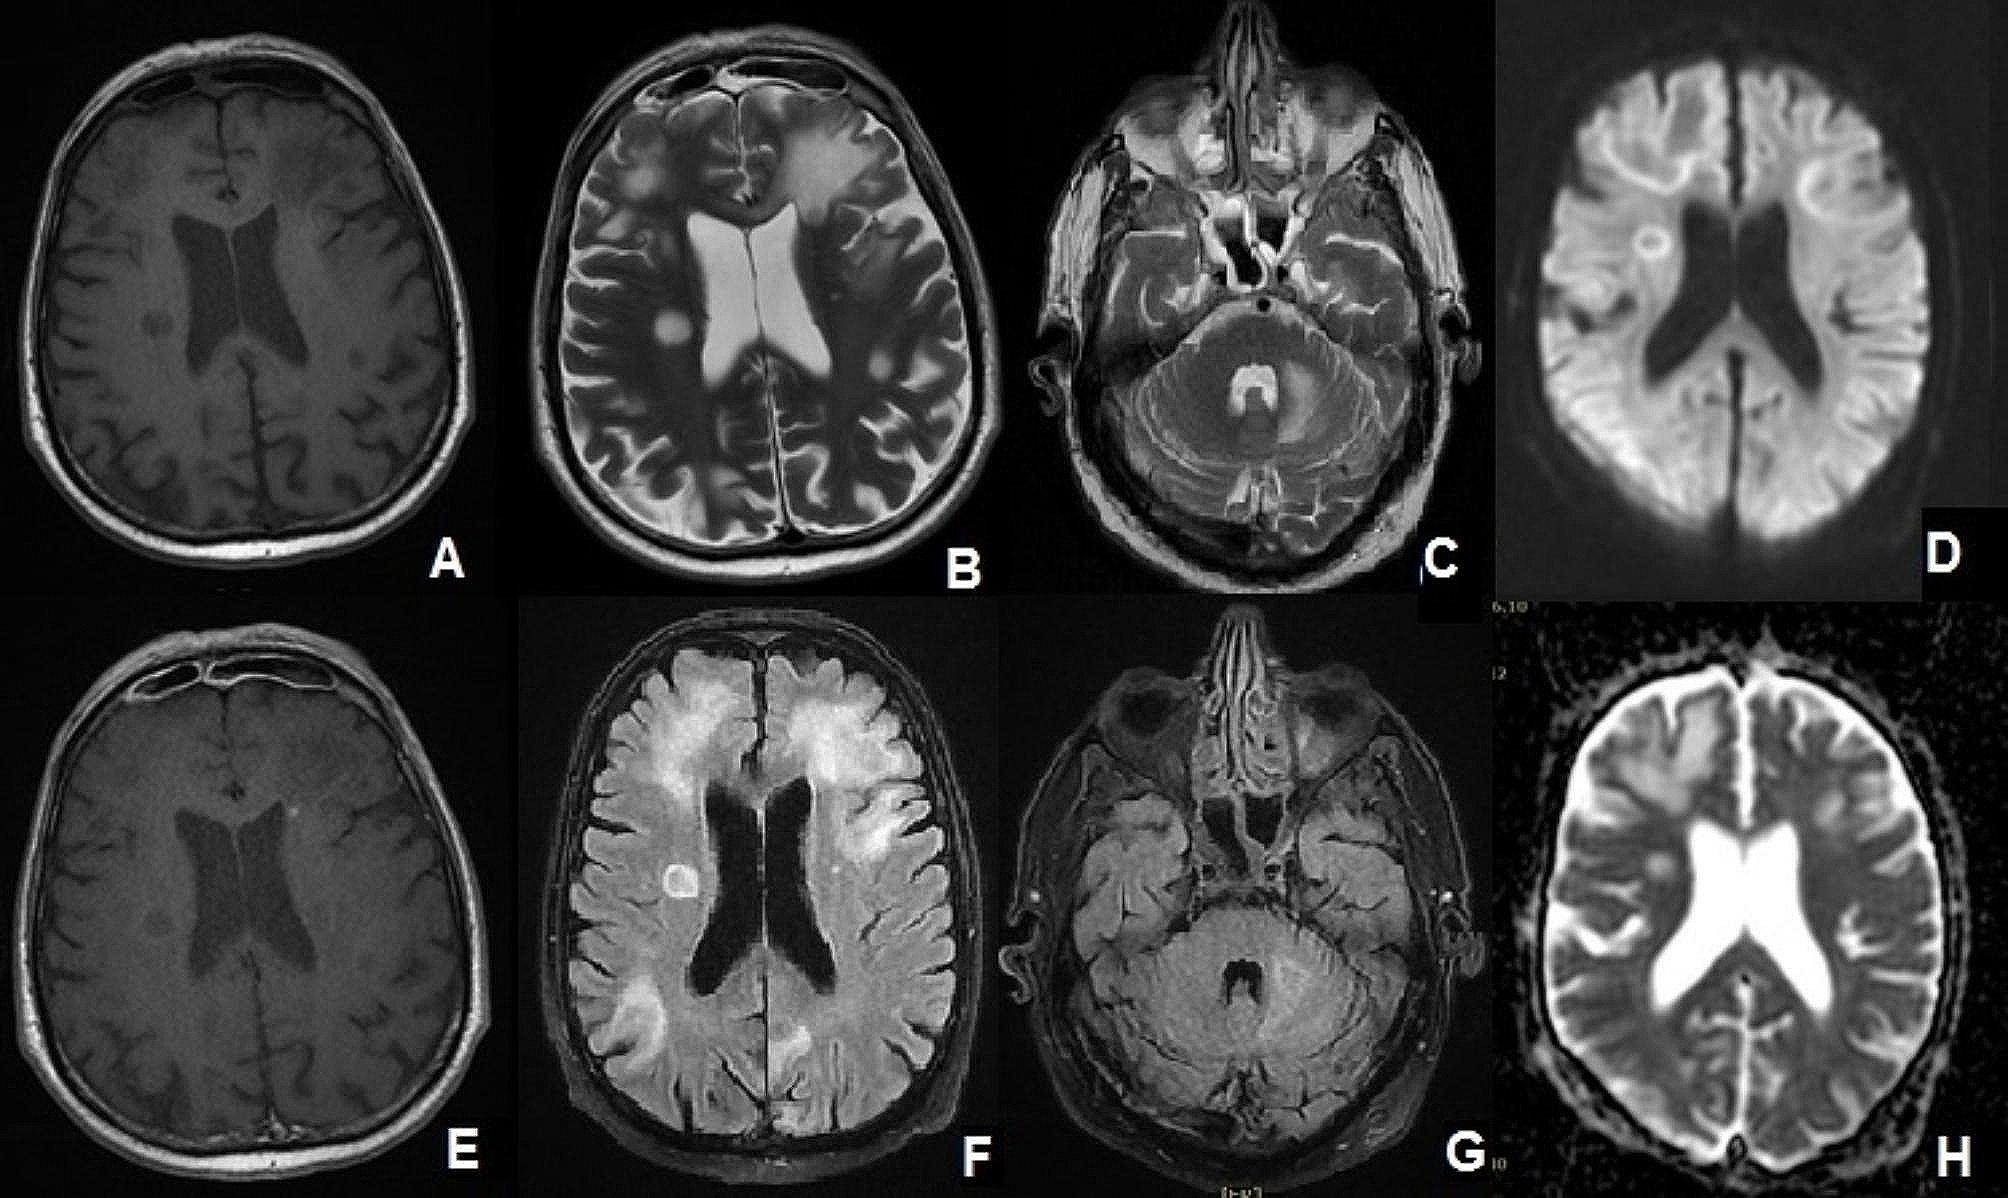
Brain MRI +/- GAD: A: in T1 sequence multiple hypointense and well-defined lesions in frontal, parietal, basal ganglia are seen. B, C, F, and G: T2/FLAIR sequences shows multiple hyperintense lesions in frontal, parietal, occipital, basal ganglia and cerebellum with sharp borders toward gray matter contrasting with ill-defined borders toward white matter. Subcortical lesions involve U-fibers. D, H: In DWI and ADC sequences peripheral restriction in noted. E: In post Gadolinium injection sequences; hazy peripheral punctate enhancement in frontal region is present. Evidence of mucosal thickening in bilateral paranasal sinuses in favor of mucormycosis sinusitis also seen (proved by pathological biopsy report)
